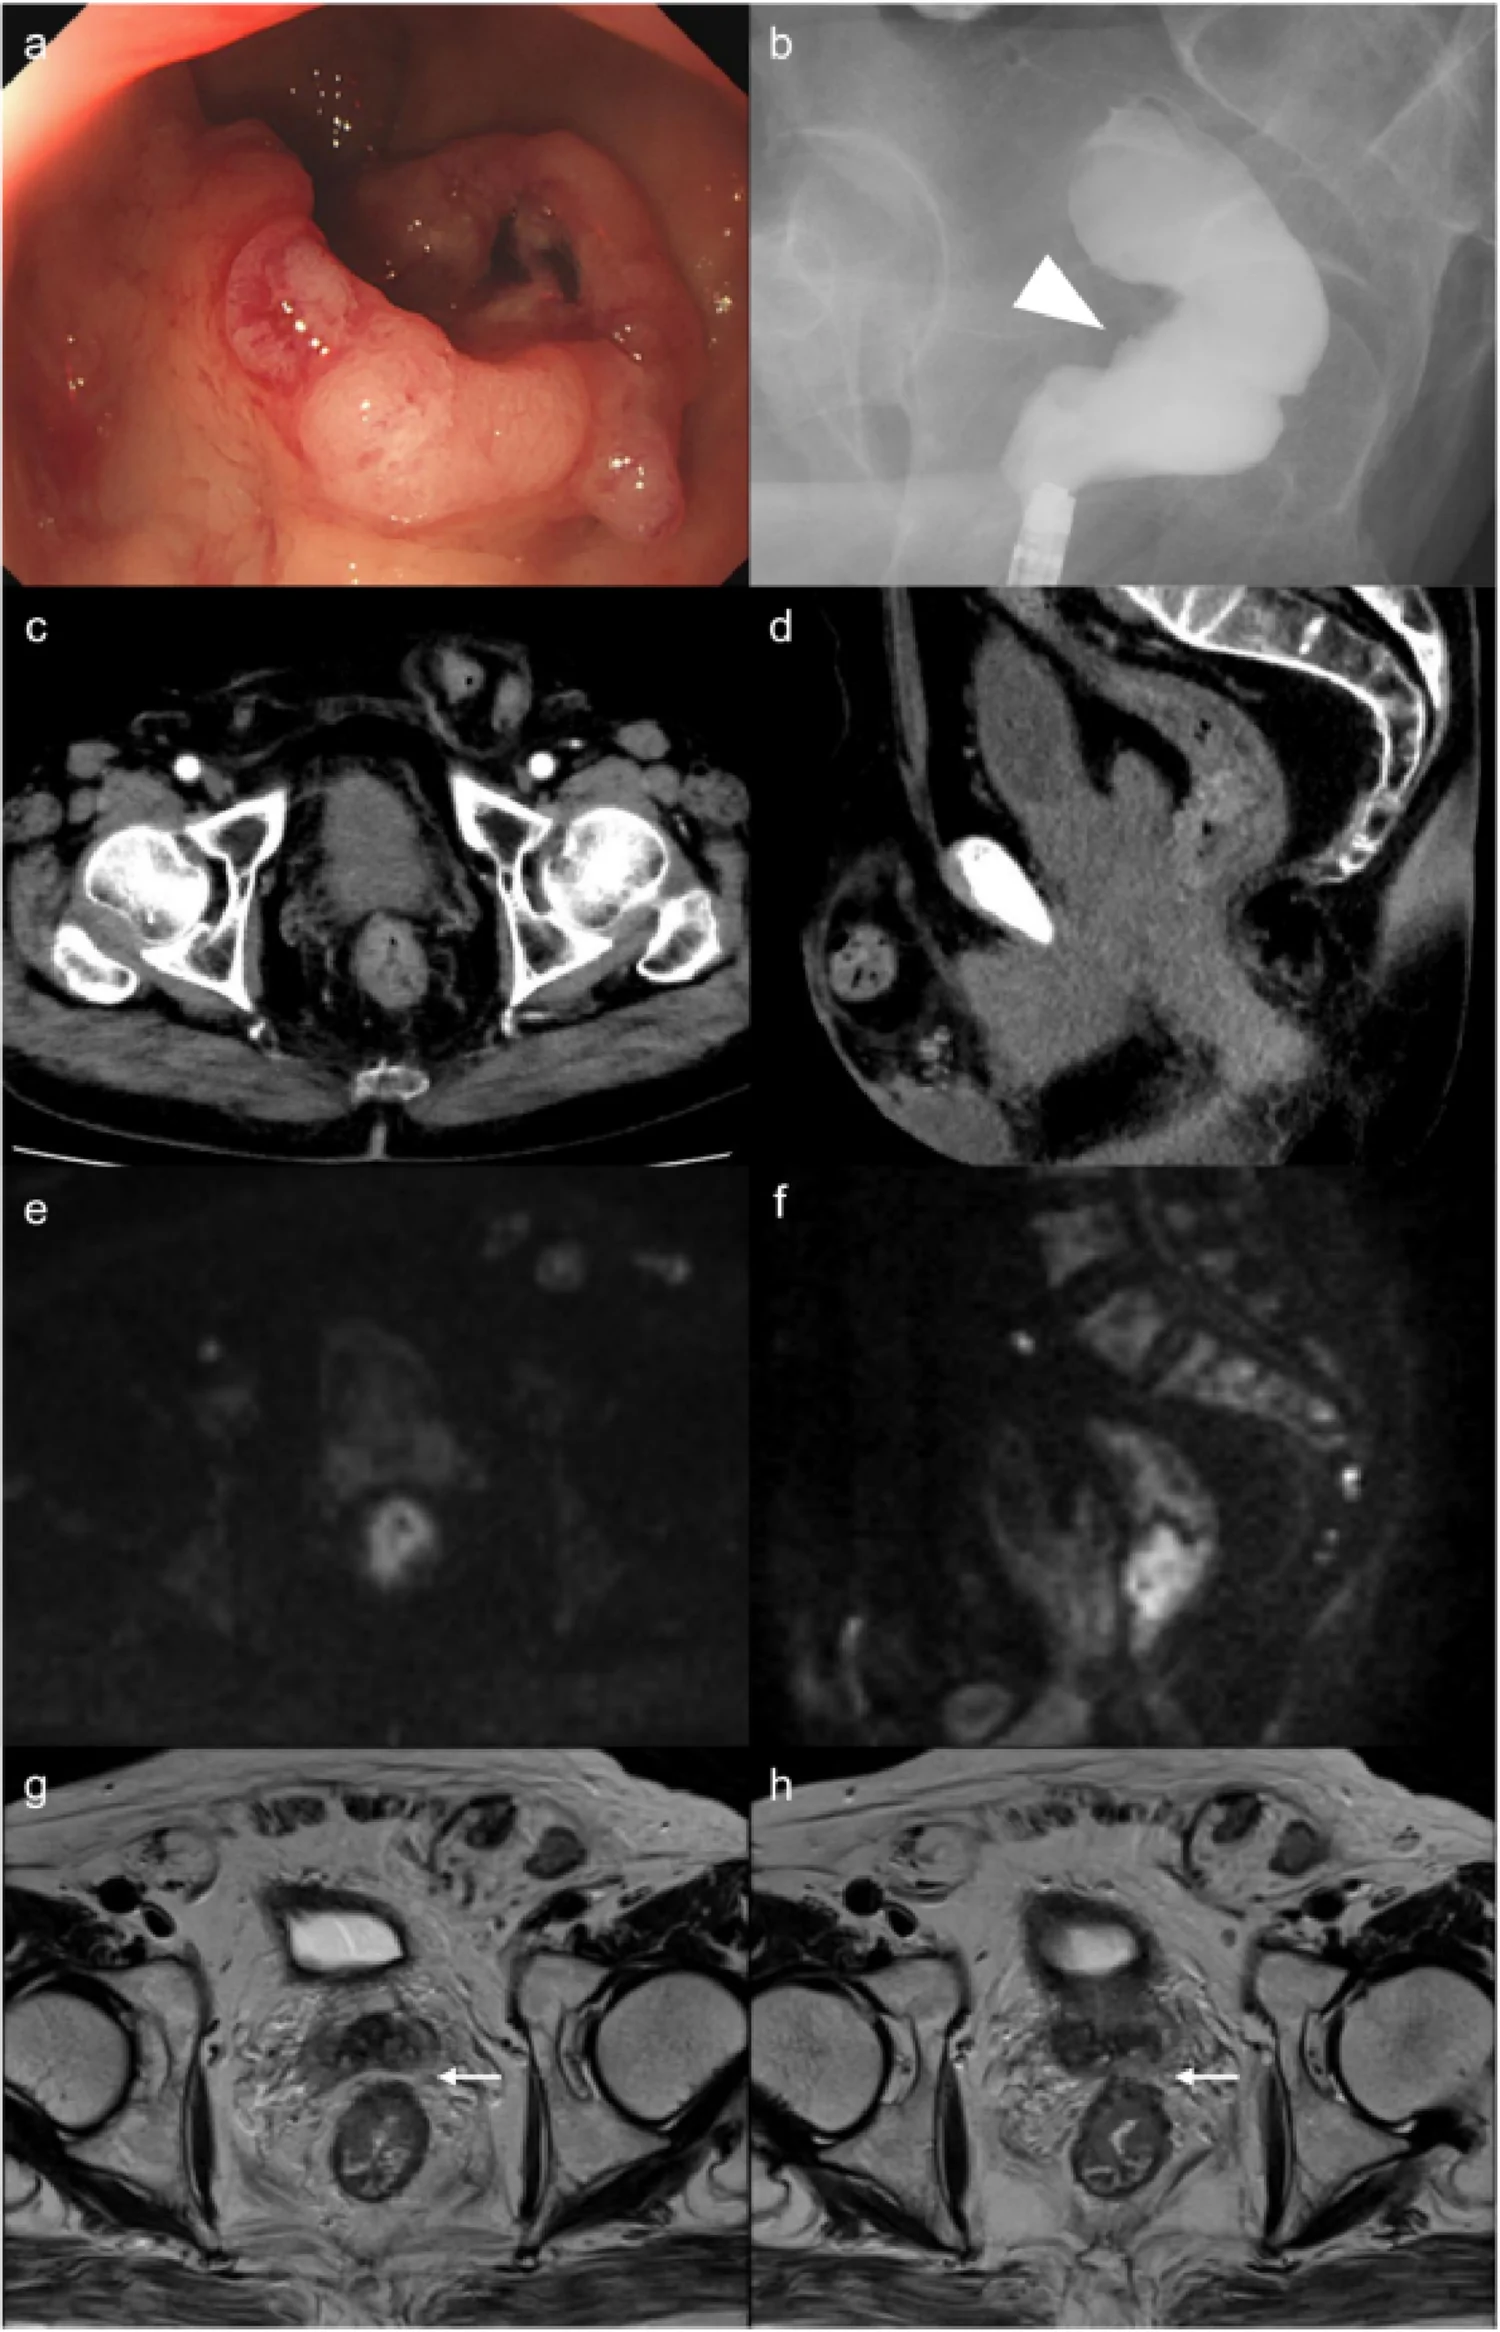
Preoperative endoscopic and radiologic findings. Colonoscopy showed a type 2 tumor in the lower rectum, 3 cm from the dentate line (a). Gastrografin enema showed irregular deformity of the anterior wall of the lower rectum (arrowhead) (b). Contrast-enhanced computed tomography (CT) showed irregular wall thickening with enhancement in the lower rectum (c, d). Magnetic resonance imaging (MRI) showed a high signal intensity area on diffusion-weighted imaging corresponding to the lesion seen on CT (e, f). Axial T2-weighted MRI showing partial loss of the muscularis propria on the right anterior wall and direct tumor abutment to the perirectal fat, without definite invasion of the external anal sphincter or levator ani muscle. Arrows indicate the site of closest proximity to the mesorectal fascia, suggesting a potentially close circumferential resection margin (g, h)
